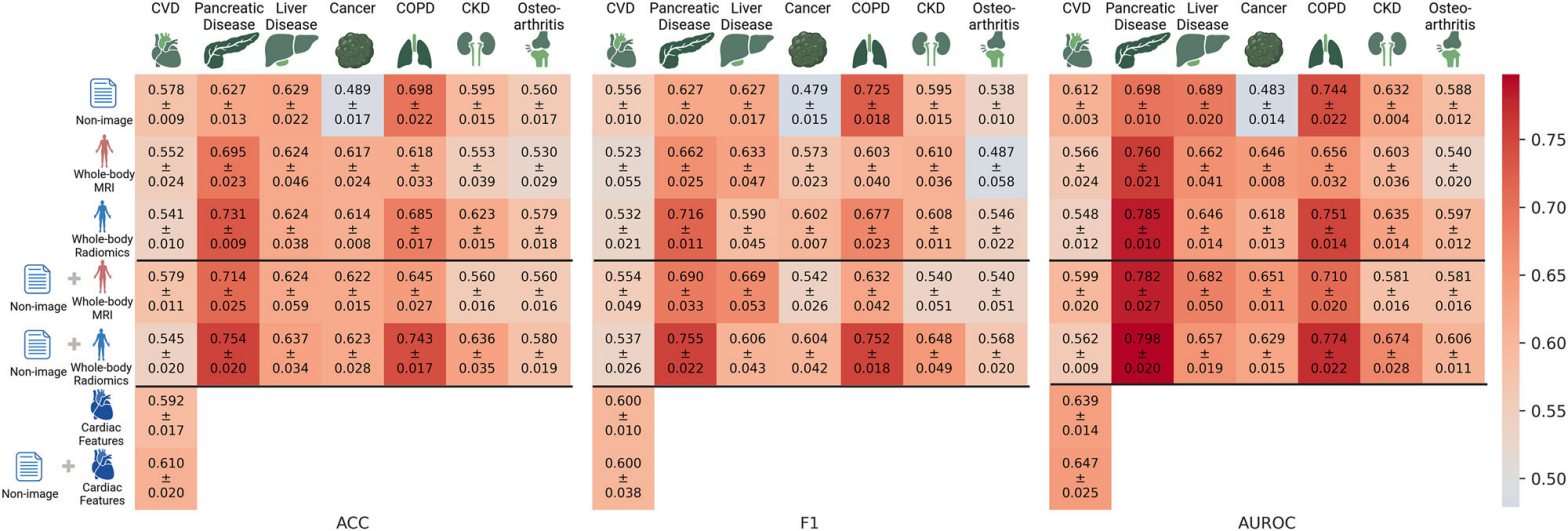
Results of 3-year preclinical risk assessment for cardiovascular disease (CVD), pancreatic disease, liver disease, cancer, chronic obstructive pulmonary disease (COPD), chronic kidney disease (CKD), osteoarthritis. The rows represent the data modality, in order: non-image, whole-body MRI, whole-body radiomics extracted from whole-body MRI, non-image with whole-body MRI, non-image with whole-body radiomics, cardiac features^30^, and non-image with cardiac features. Created in BioRender. Seletkov, D. (2025) https://BioRender.com/m09n1fh.
